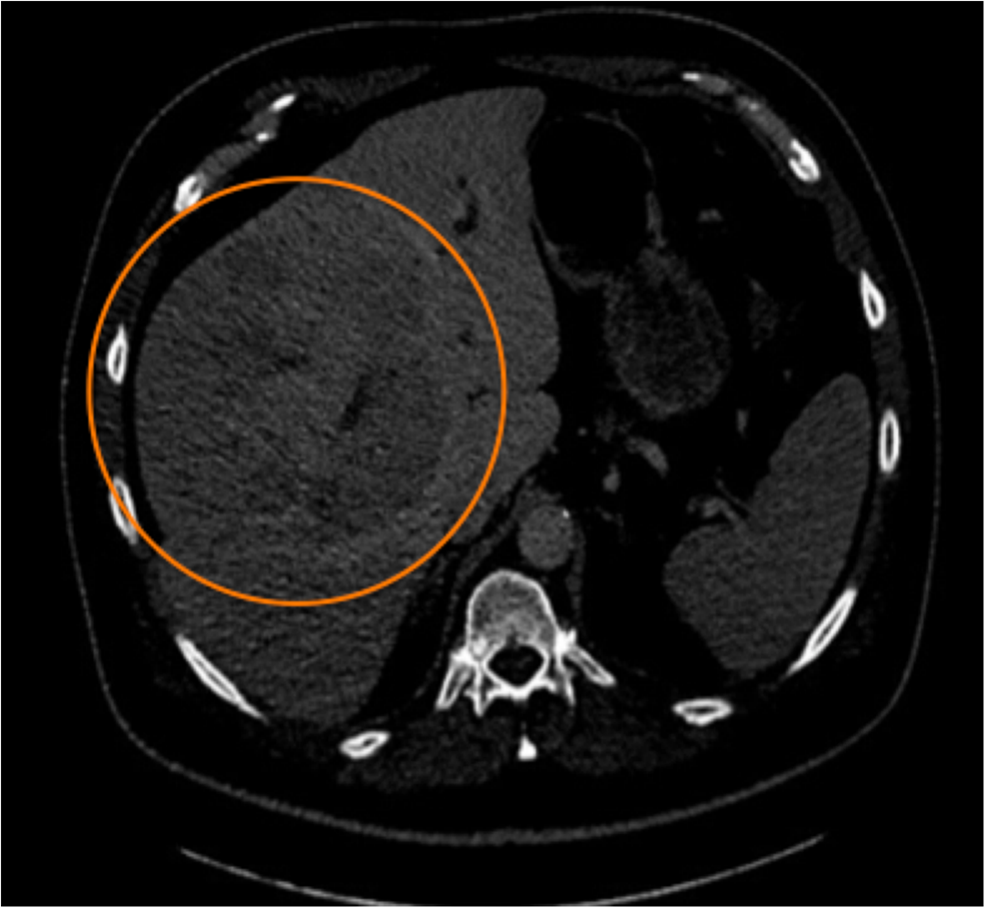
Contrast-enhanced CT of a male patient with a 14-cm HCC (orange circle) with a tumor volume of 1695 cm^3^ and inadequate FLR (560 cm^3^) for surgical resection
